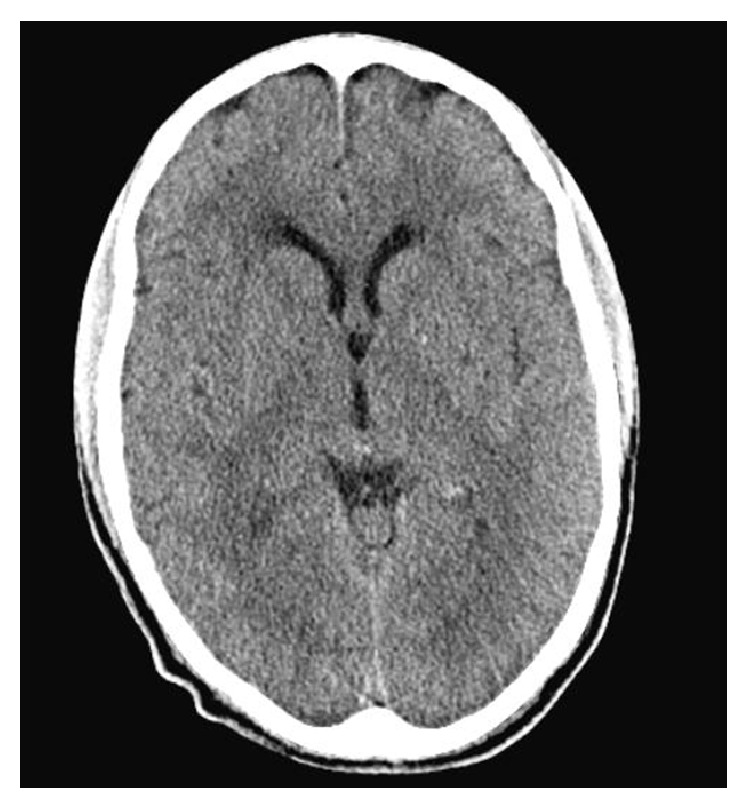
Reveals normal ventricular size on a CT head w/o contrast.
